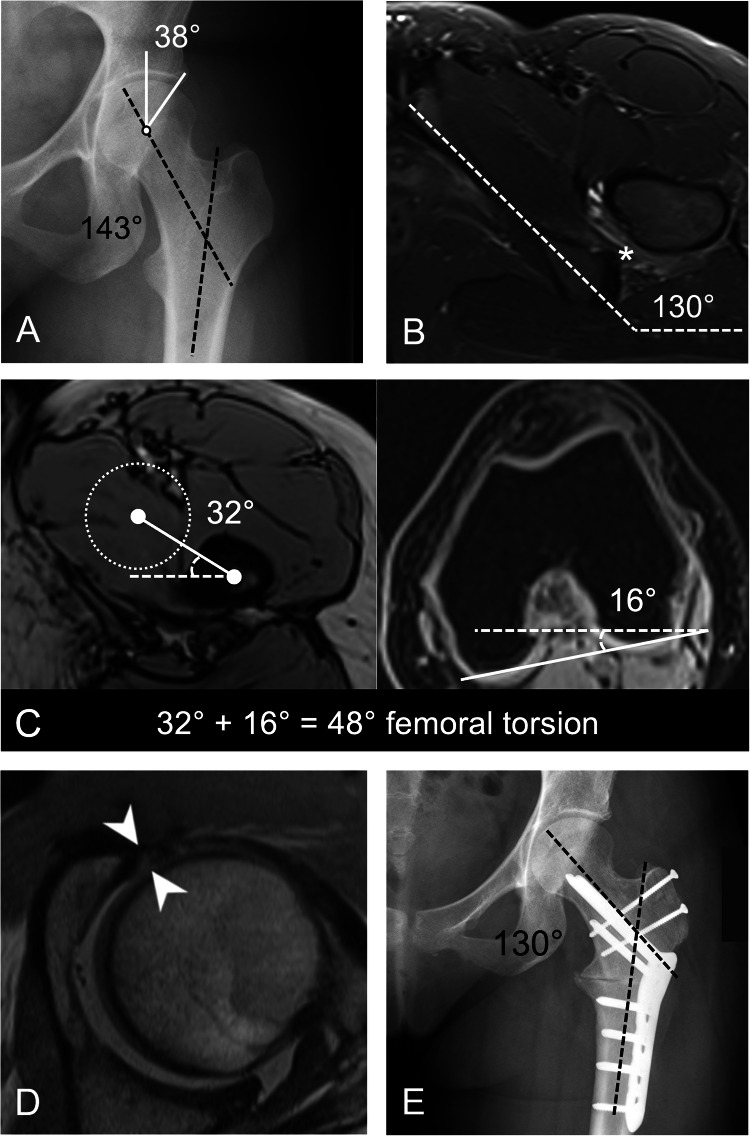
AP pelvic radiograph of a 25-year-old female patient with left buttock pain and positive posterior impingement test. Diagnostic imaging revealed (A) a valgus deformity (neck shaft angle of 143°) and acetabular overcoverage (LCE 38°). The patient underwent subsequent hip MRI which showed (B) increased ischial angle and edema in the quadratus femoris muscle (asterisk) on axial STIR MR-image, (C) increased femoral torsion (48°) measured on axial T1-w volume-interpolated breath-hold examination DIXON MR-images. D The axial oblique proton-density weighted turbo spin echo MR arthrogram showed an intrasubstance tear of the anterosuperior labrum (arrowheads) without concomitant cartilage damage. Overall this led to the clinical diagnosis of IFI. The patient underwent subsequent (E) surgical hip dislocation with additional intertrochanteric varisation-derotational osteotomy with good relief of symptoms 6 months after surgery
